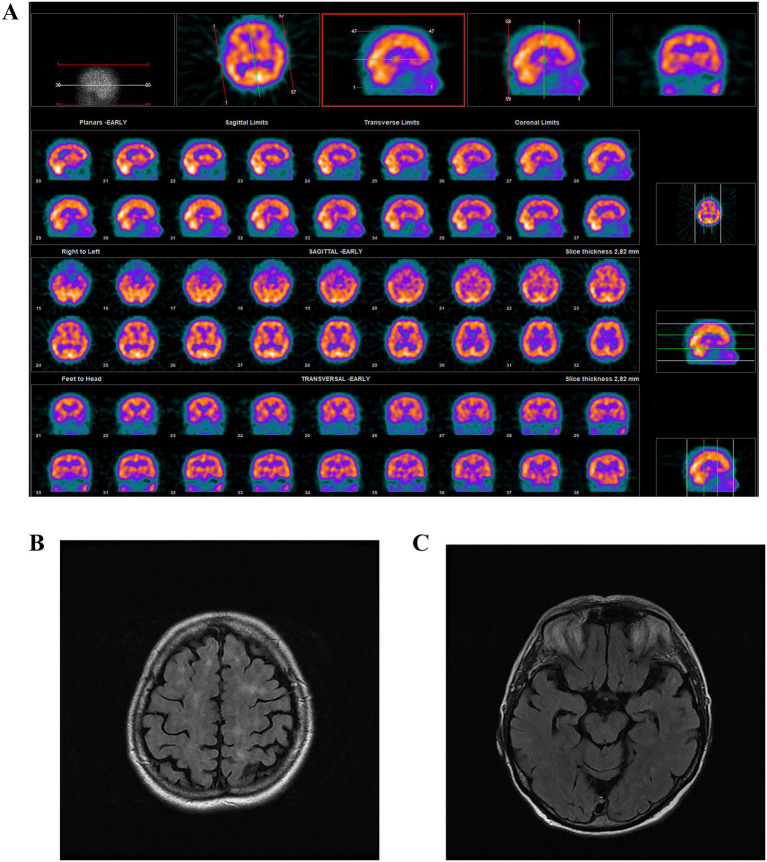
SPECT imaging (A) showed diffusely reduced cortical activity, with borderline findings suggestive of global cortical hypometabolism. MRI, from 2015 (B,C), showed significant cortical and subcortical atrophy, predominantly in the temporal lobes and hippocampi, with focal chronic ischemic white matter lesions resulting from microangiopathy. Periventricular hyperintensities on T2/FLAIR MRI, categorized according to vascular involvement.
